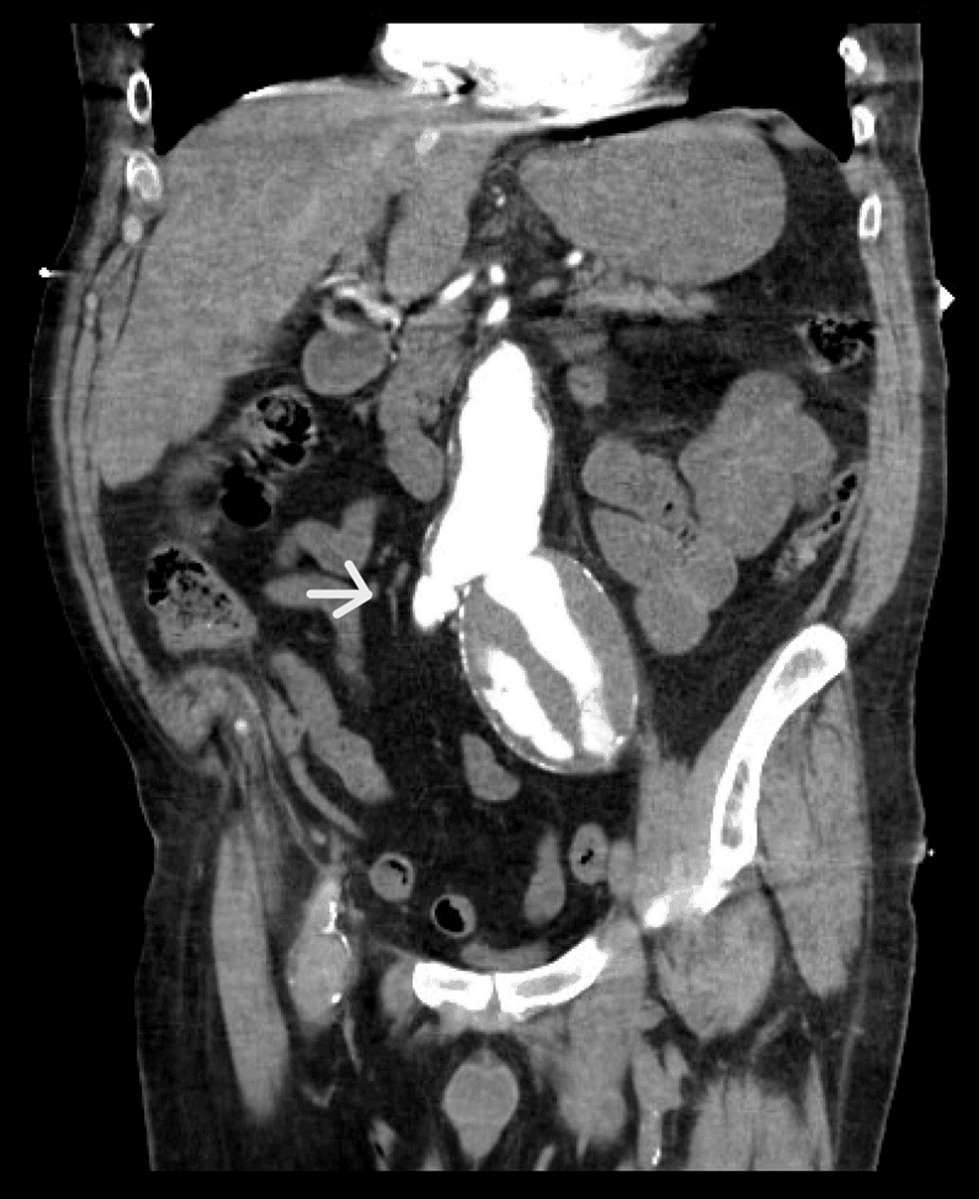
CT with IV contrast demonstrating contrast filling the iliac artery and vein (arrow)
